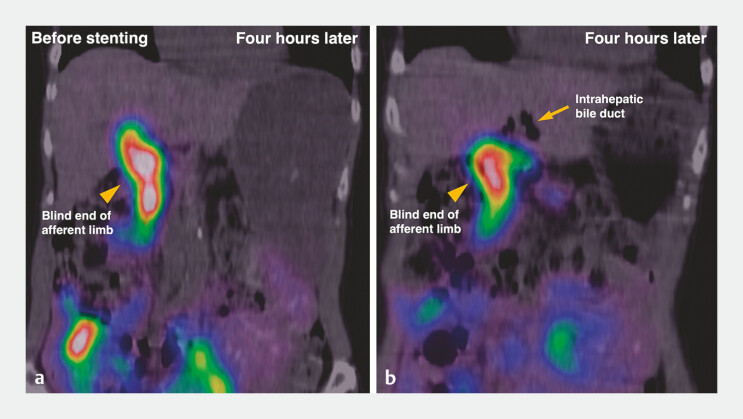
Hepatobiliary scintigraphy before stenting, on delayed imaging 4 hours later.
